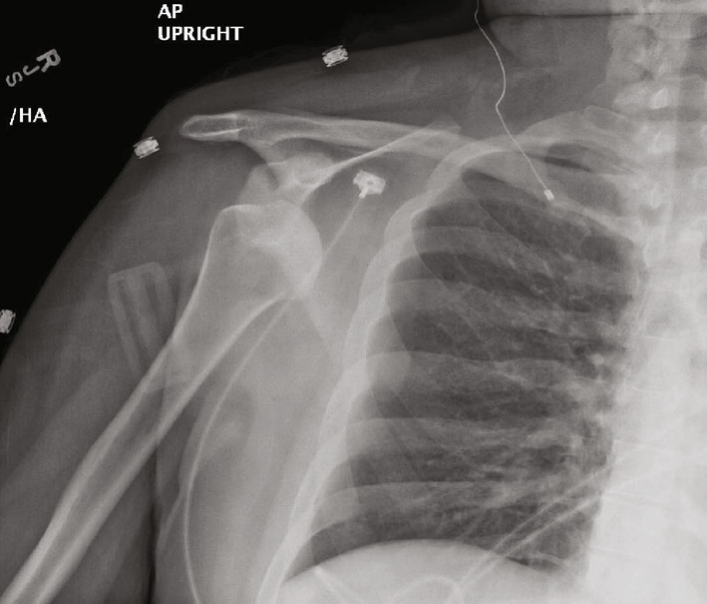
AP right shoulder, prereduction (case 1). Typical radiographic appearance of an anterior glenohumeral dislocation.
